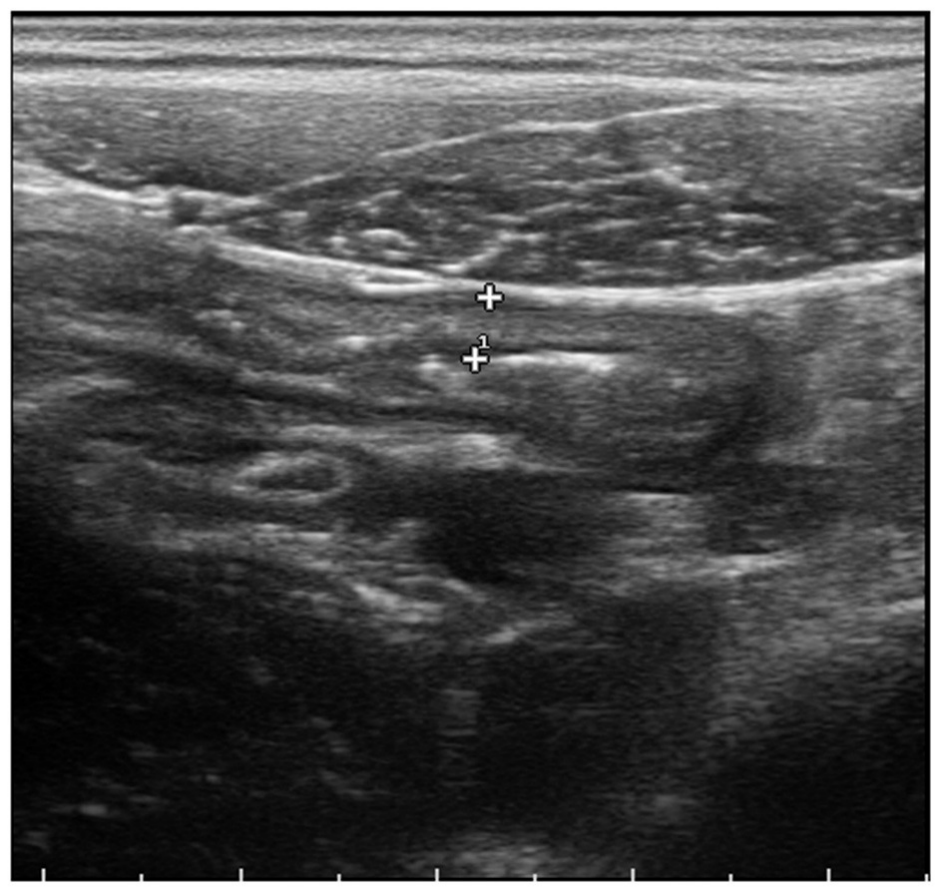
Abdominal ultrasound showing transmural thickening of the terminal ileus.
